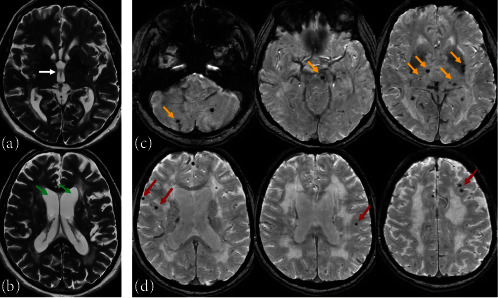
The examples of brain MRI finding of patients with ESUS. (a) T2 WI, white arrow indicates dilated third ventricle. (b) T2 WI, green arrows indicate the dilatation of the lateral ventricles due to central brain atrophy. (c) SWAN MRI, orange arrows indicate deep microbleeds. (d) SWAN MRI, red arrows indicate subcortical microbleeds.
